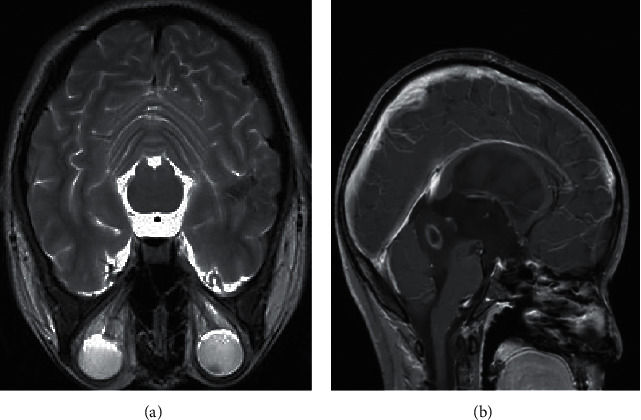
MRI image of brain structure. Image (a) is in the coronal plane, and (b) is in the sagittal plane.
